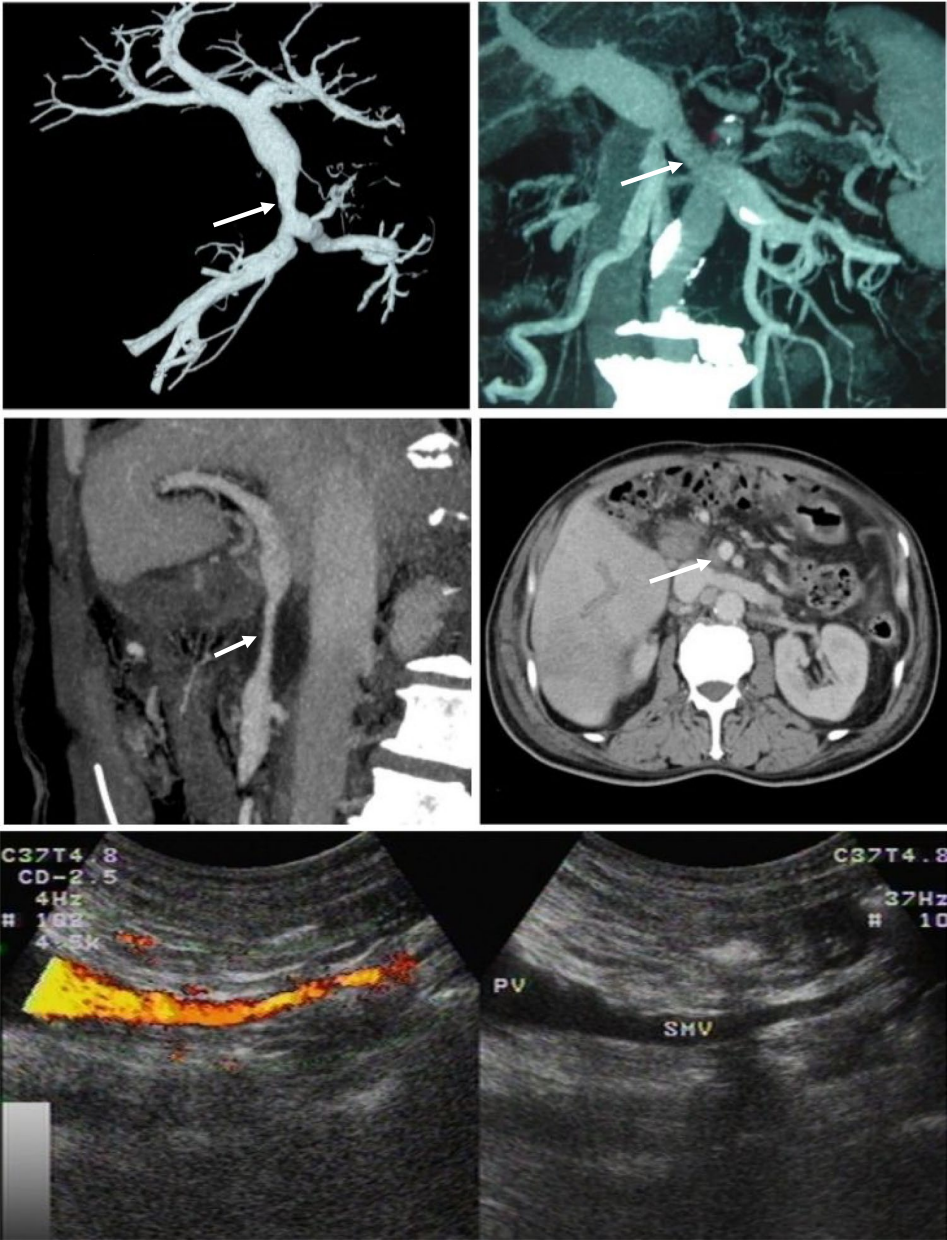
Images of the reconstructed portal vein and/or superior mesenteric vein. a Postoperative 1-month enhanced computed tomography (CT) reconstruction image of ligamentum teres hepatis (LTH) patch graft for portal-superior mesenteric vein (PSMV) reconstruction; b postoperative 3-month enhanced CT reconstruction image of LTH interposition graft for superior mesenteric vein (SMV) reconstruction; c postoperative 6-month coronal CT images of LTH patch graft for PSMV reconstruction; d postoperative 1-year sagittal CT images of LTH patch graft for PSMV reconstruction; e postoperative 2-week doppler ultrasound images of LTH graft for reconstruction
